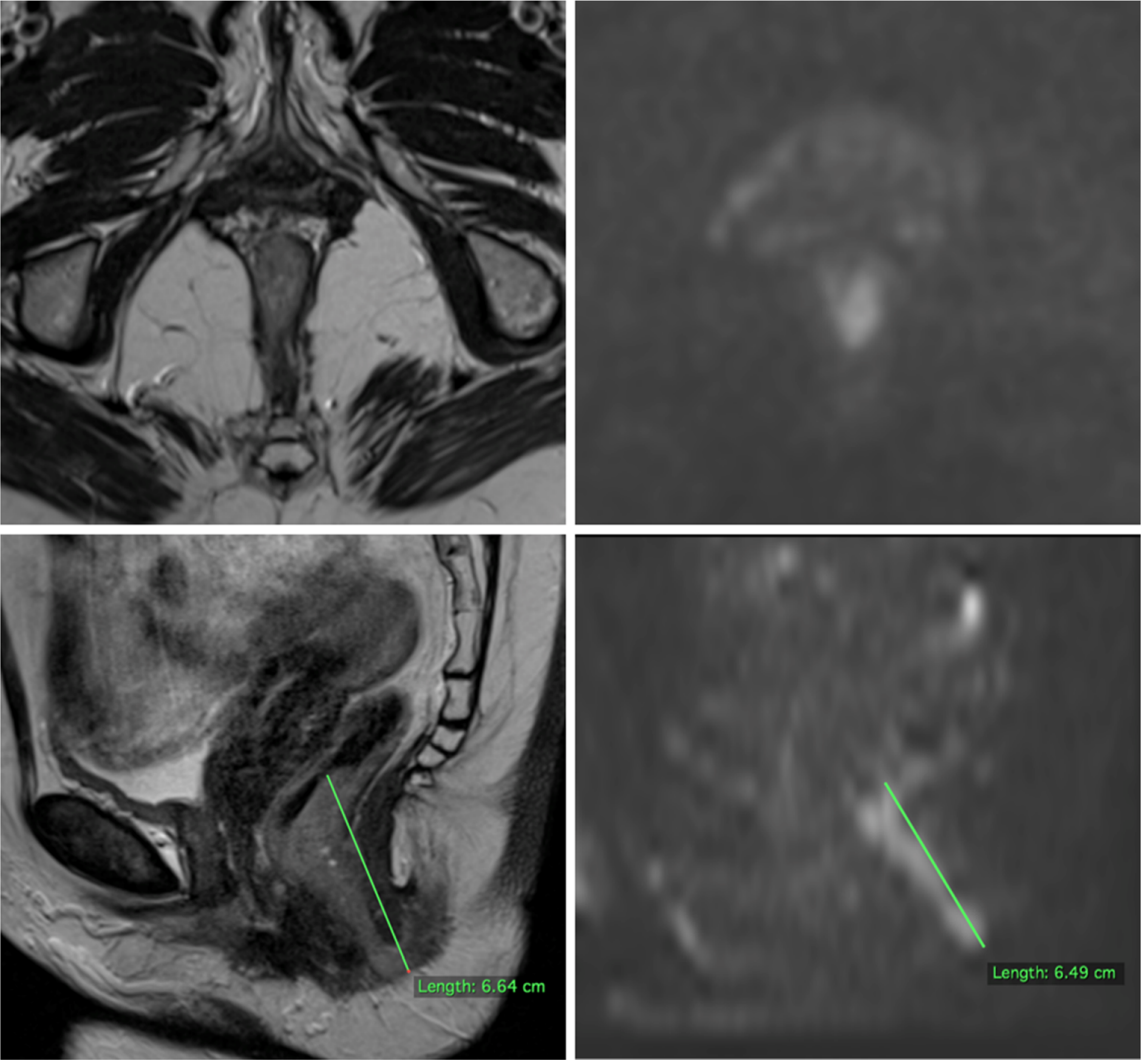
Anorectal SCCA. High-resolution T_2_-w axial-oblique image at the level of the anorectal junction (top left): lesion contouring is challenging considering the suboptimal contrast resolution between tumour and adjacent mucosa. Same-level high *b*-value axial DWI (top right): the hyperintense lesion can be clearly outlined against the suppressed signal of surrounding healthy tissue. T_2_-w sagittal image used for MTD measurement (bottom left). Corresponding sagittal reformat of high *b*-value DWI, also used for MTD measurement (bottom right)
